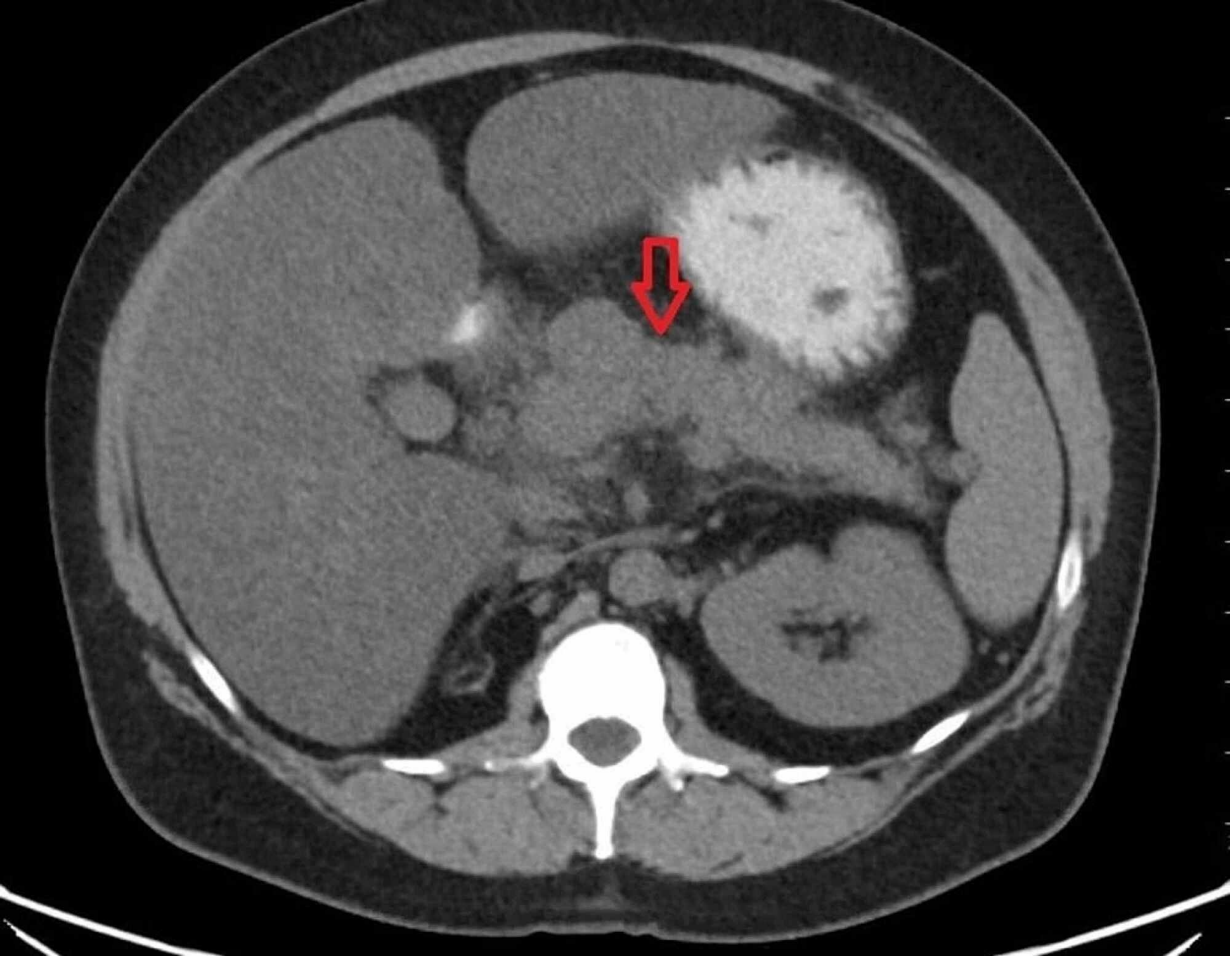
CT scan of abdomen showing pancreatitis and peripancreatic fluid collections
